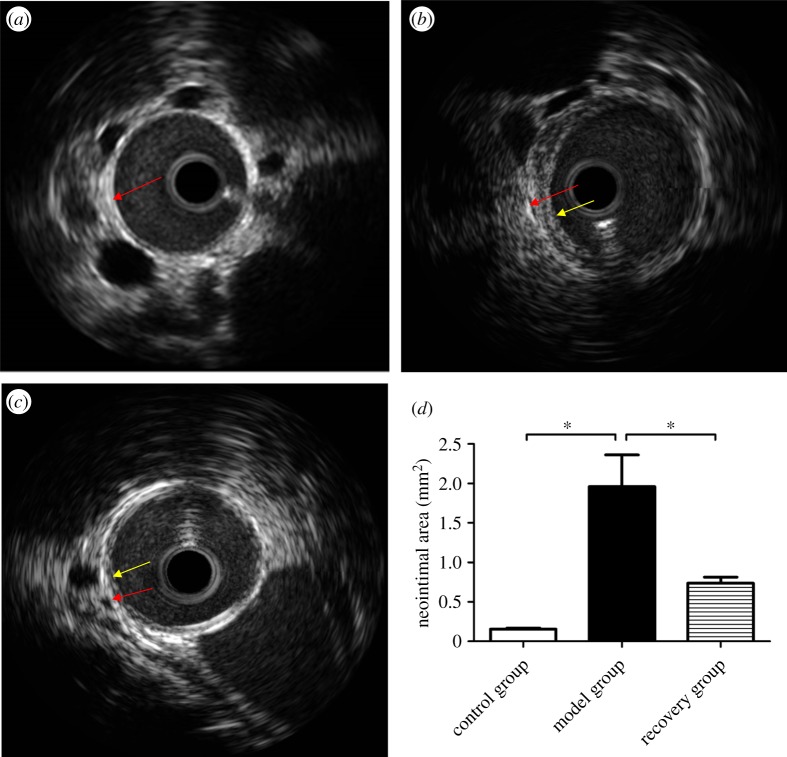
Intravascular ultrasound of rabbit atherosclerosis. Intravascular ultrasound images show representative pictures of rabbit abdominal aorta in control, model and recovery groups, respectively (*a*, *b* and *c*). Obvious neointima was observed in the model group and little neointima was observed in the recovery group, whereas no neointima was observed in the control group. The mean neointimal area was measured and analysed (*d*). The red and yellow arrows indicate the intima and the neointima, respectively. Data were reported as means ± s.d. and among three-group comparisons were performed by ANOVA, *n* = 4. p* < 0.05. A *p*-value of less than 0.05 was considered significant.
